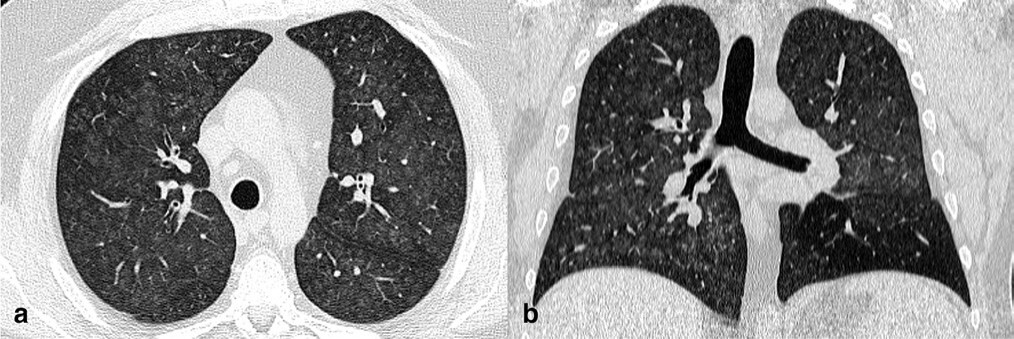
CT images of a 58-year-old male patient with confirmed COVID-19 pneumonia (γ variant). Axial (a) and coronal reformatted (b) images showed diffuse lung involvement with ill-defined centrilobular GGO and subpleural sparing. GGO, ground-glass opacity.
